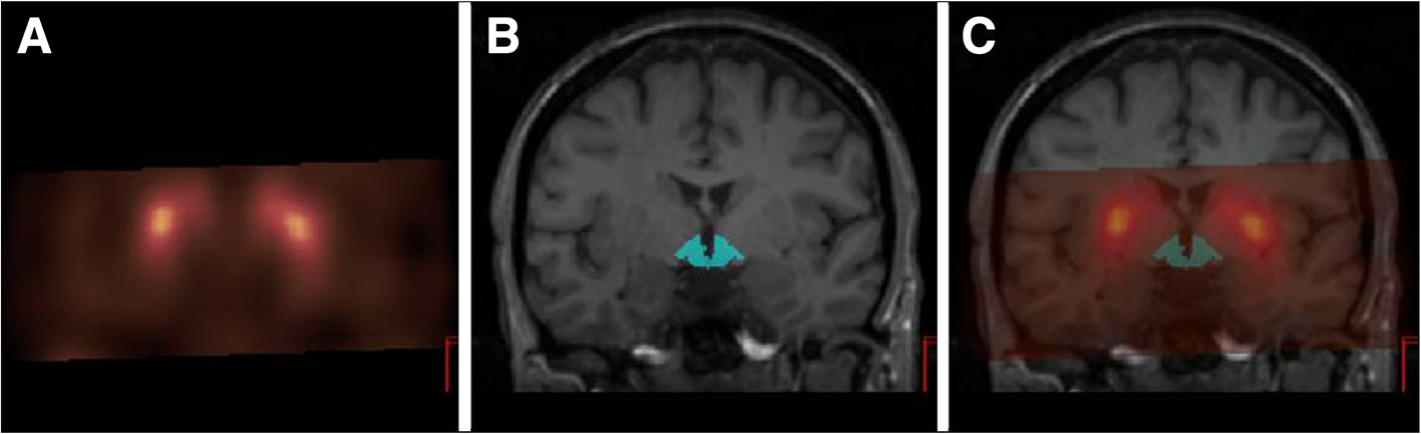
SPECT and MRI of the hypothalamus. (A) Coronal SPECT image of a healthy subject 2 h after injection of approximately 115 MBq [^123^I]FP-CIT at the level of the hypothalamus. (B) Coronal T1-weighted MRI image of the same subject with ROI drawn on the hypothalamus. (C) Coregistered SPECT and T1-weighted MRI image with ROI drawn on the hypothalamus. The SPECT images are color encoded for low (black) to high activity (yellow).
